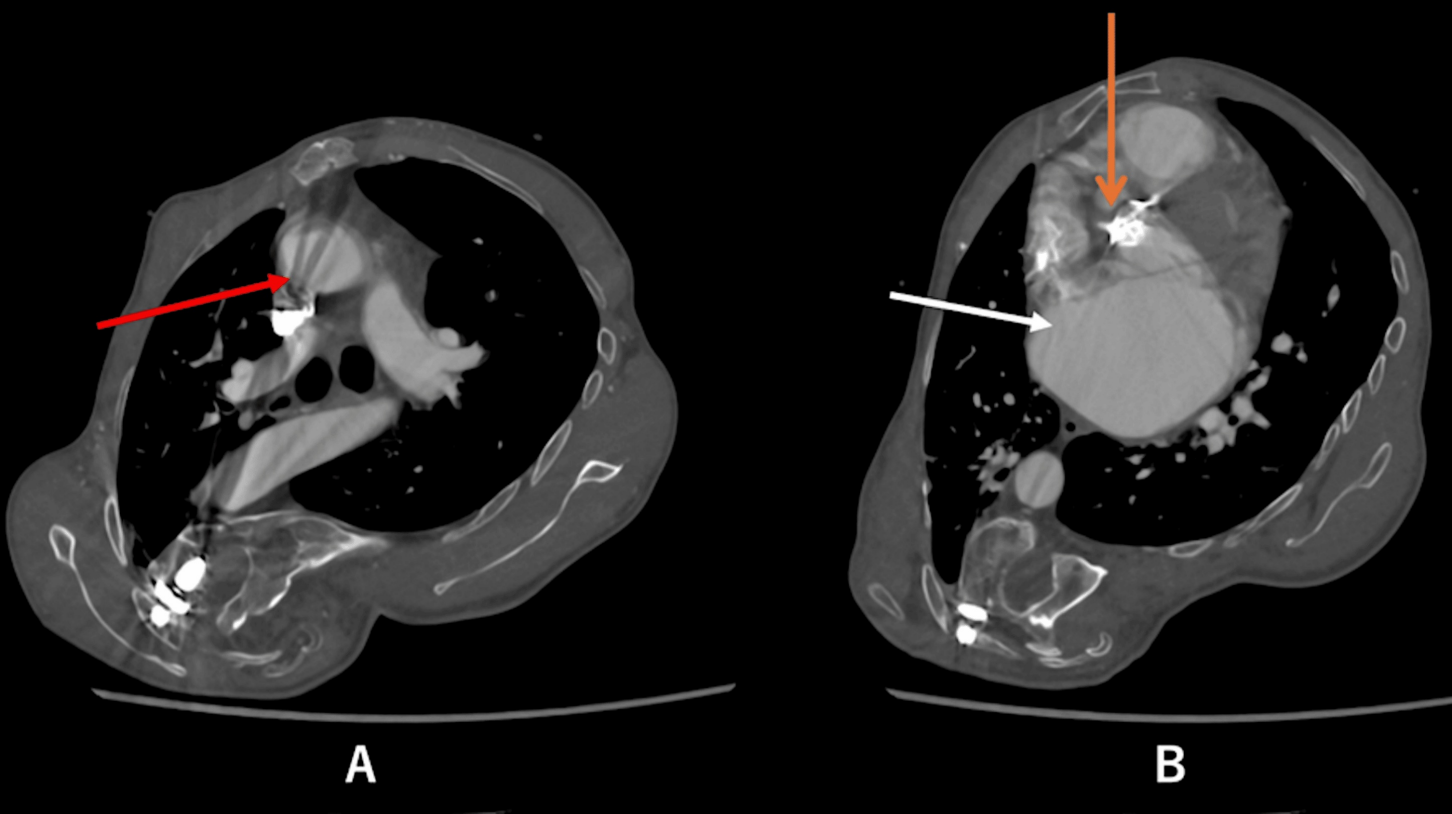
Axial CT images at the level of the second ICS (A) and fourth ICS (B) The orange arrow indicates the trajectory of a median sternotomy, which does not provide adequate exposure for the mitral valve. The white arrow indicates the right thoracotomy approach through the fourth ICS, while the red arrow indicates the second ICS, used for dissection around the aortic graft. ICS: intercostal space
